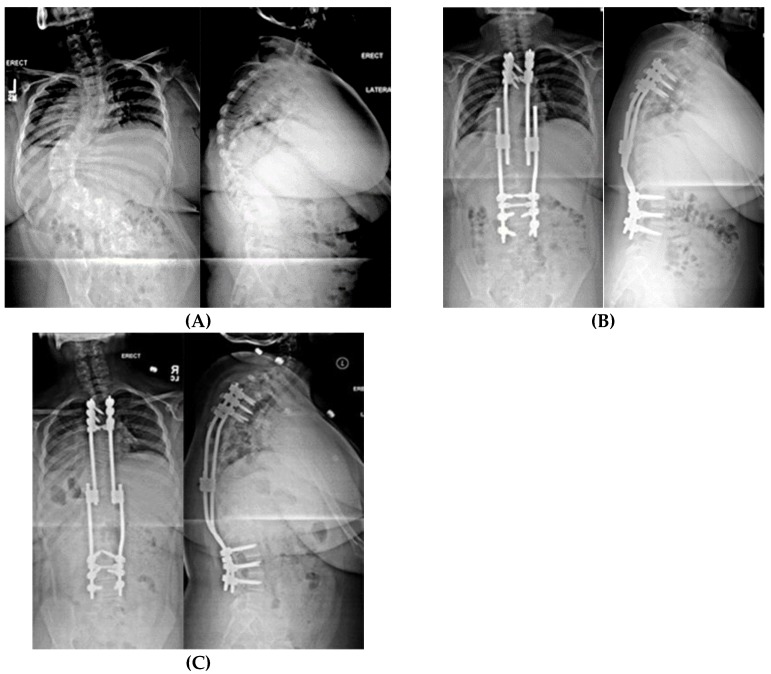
(A) AP and lateral radiographs of a 10-year-old female with PWS deletion type with 103° curve. (B) AP and lateral radiographs 1 month later, after placement of non-fusion spinal instrumentation. Curve is 57°. (C) 18 months later, after 50mm of lengthening, curve is 25°.
